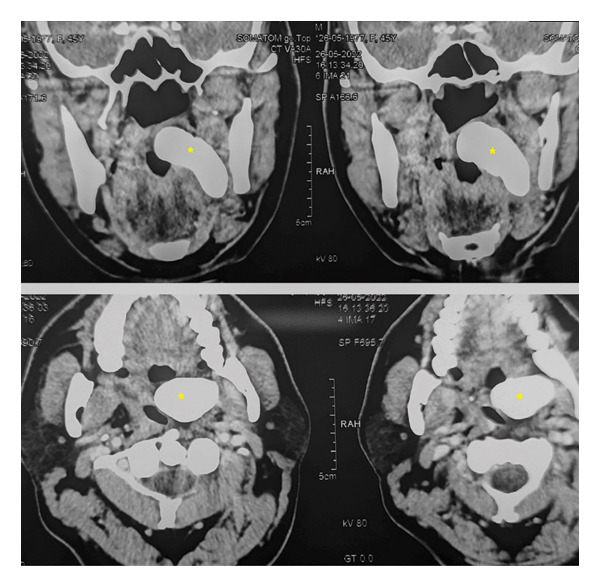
CT scan showing a large tonsillolith.
